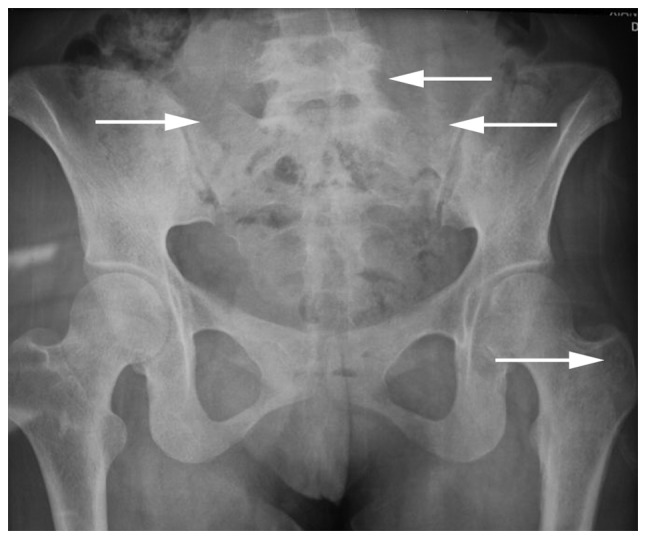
Pelvic X-ray film revealing a bone lesion (white arrows) in each sacroiliac joint and in the inferior segment of the lumbar spine. The left greater trochanter is not visible.
